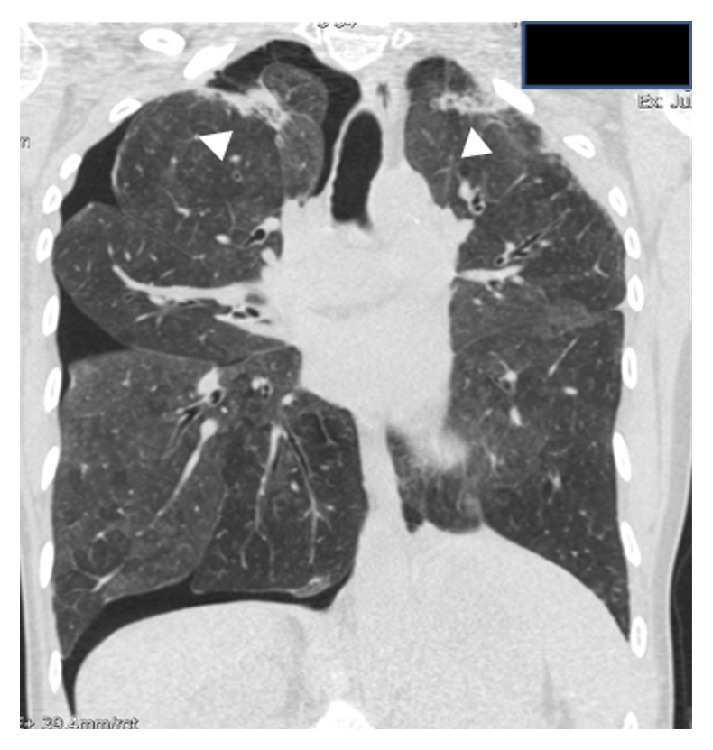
Case  2. Coronal HRCT reformatted image shows a right pneumothorax and the presence of fibrotic pulmonary infiltrates in both apical regions (arrowheads).
